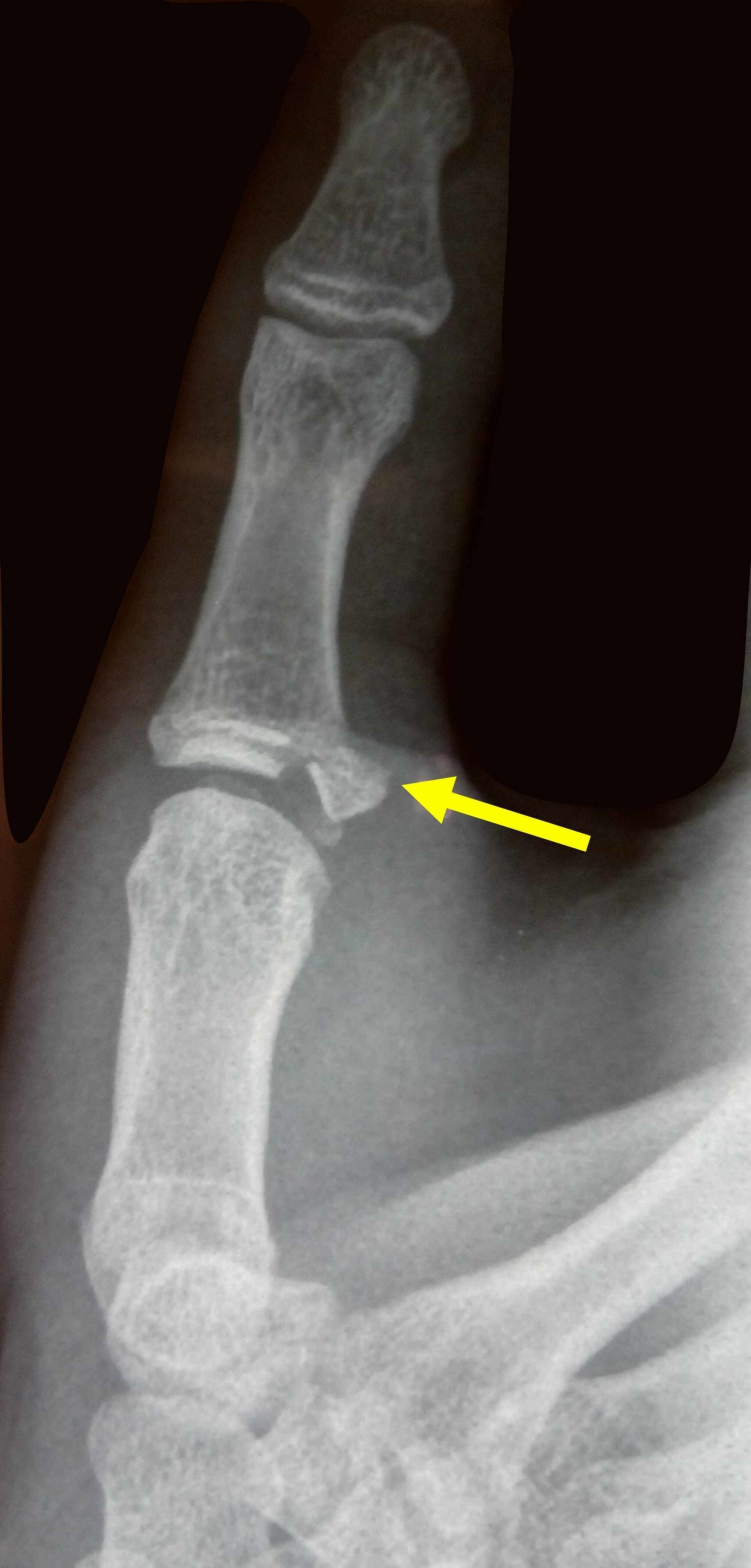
X-ray view of the right thumb of a 15-year-old boy after a fall from his bicycle The yellow arrow indicates the avulsion fracture of the attachment of the ulnar collateral ligament of the thumb metacarpophalangeal joint.
